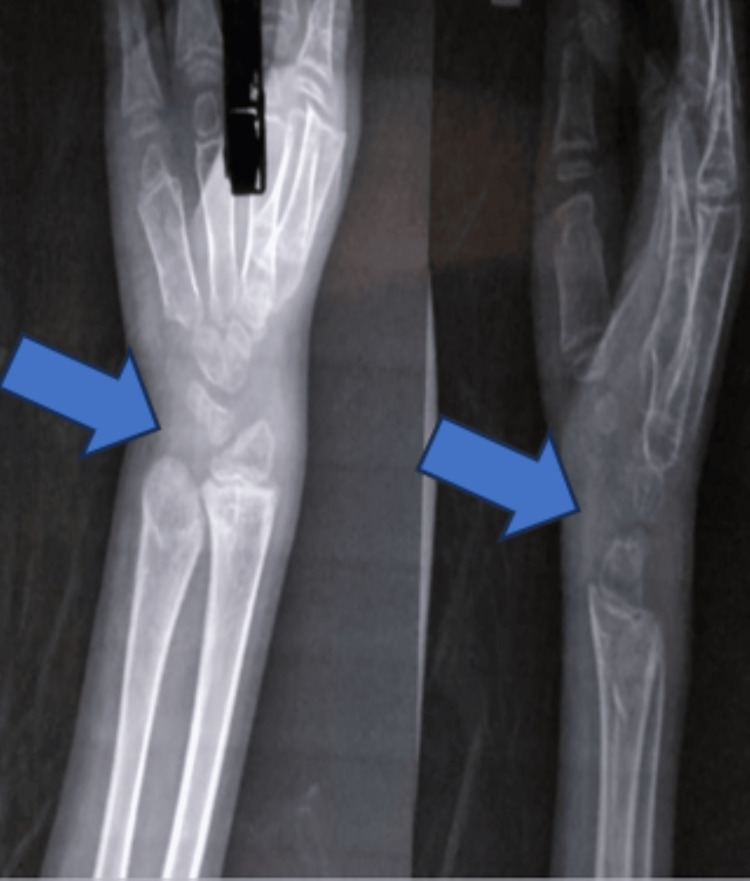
Wrist and hand X-ray findings (arrows) of the child showing features of dysostosis multiplex.
